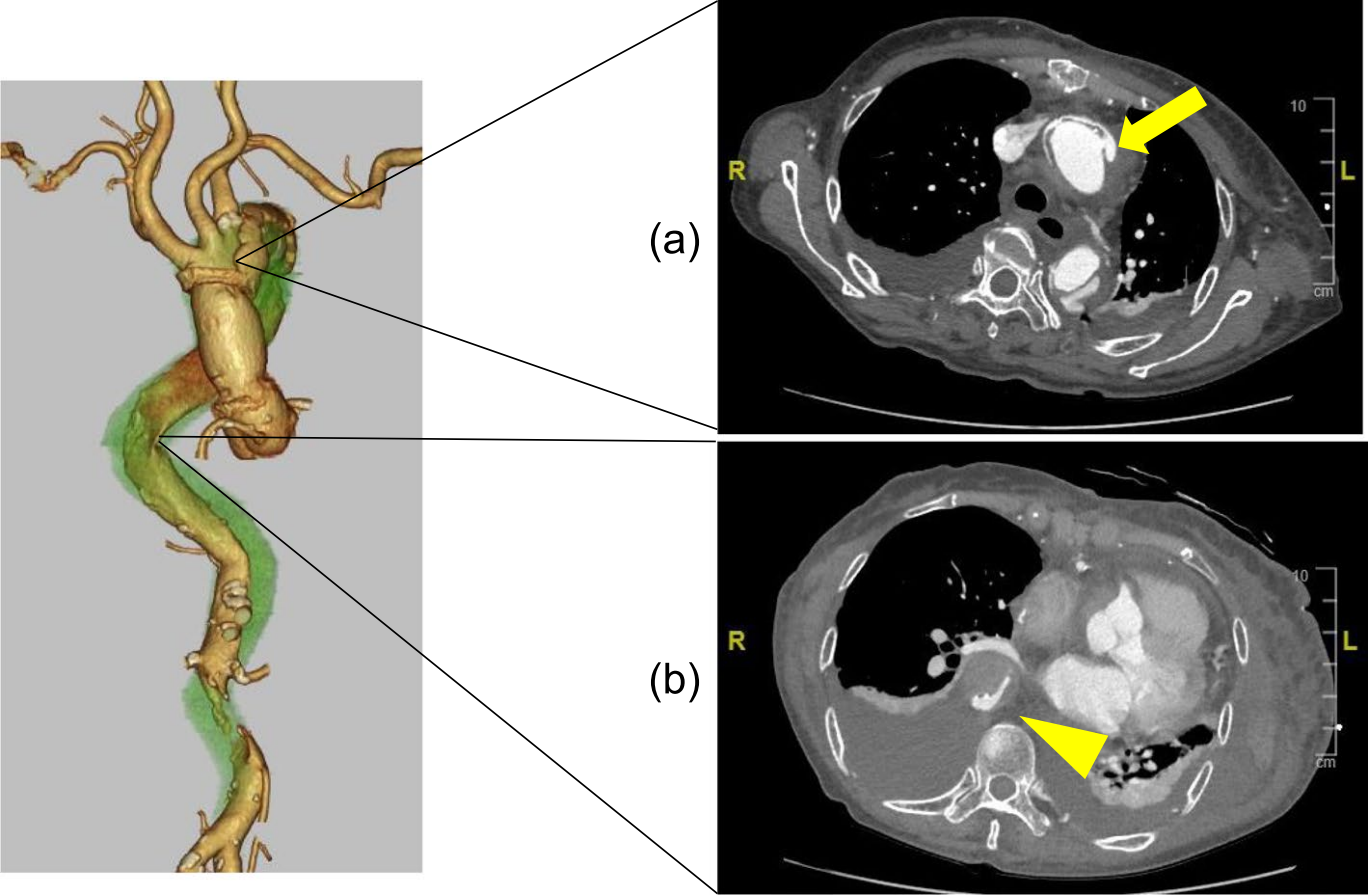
CT findings after the primary surgery. a CT shows the new entry (arrow) at the distal anastomotic site. b CT indicates stenotic true lumen and expanded false lumen at the descending aorta (arrowhead). CT, computed tomography
